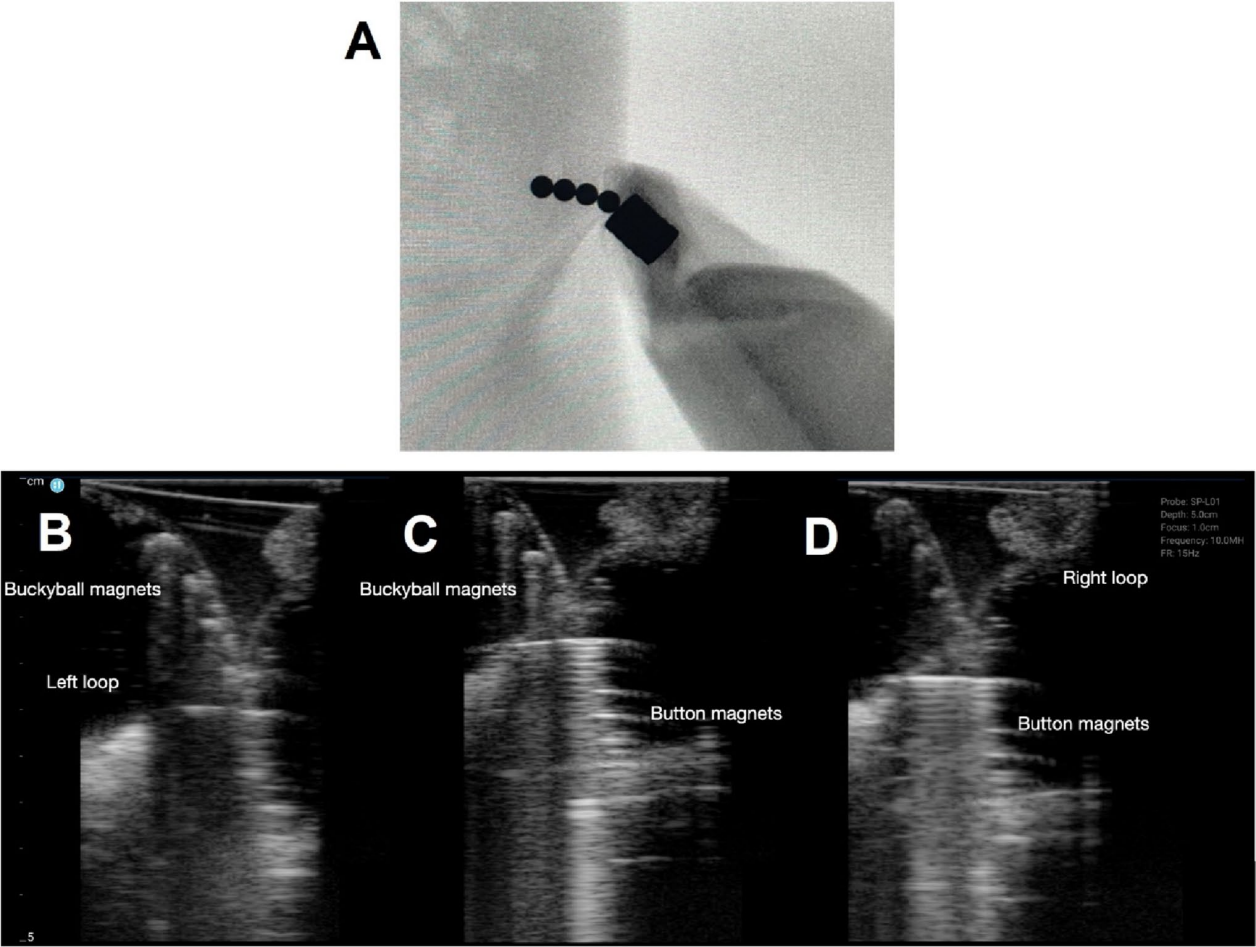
Four Buckyball magnets and a stack of button magnets in proximity. A X-ray appearance of the magnets in fluid in bowel. Ultrasound images of the 4 Buckyball magnets seen in the (B) left loop of bowel (C) tethered in the middle (with a “V” shape) and a (D) stack of button magnets seen in the right loop
